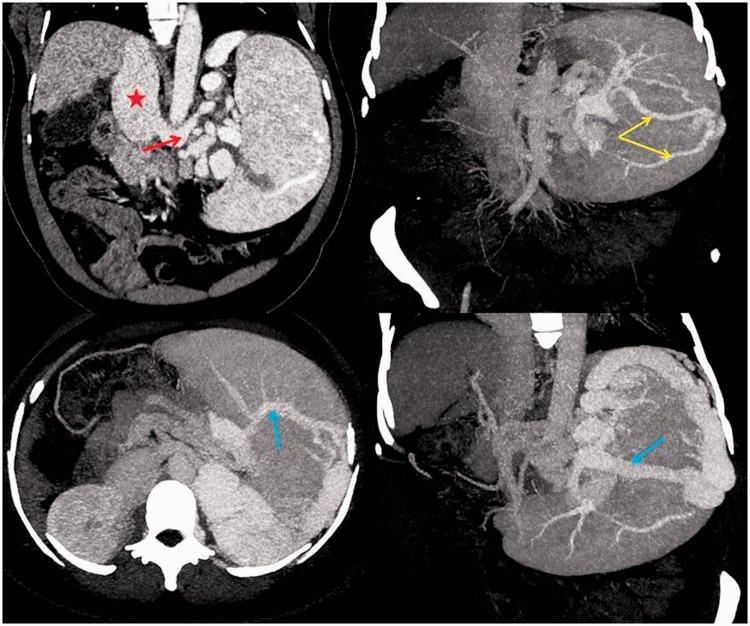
Computed tomography imaging of the abdomen with 3D reconstruction. Clockwise from left: splenorenal shunt from origin of the IVC (red star); left renal vein (red arrow) joining the splenic vein at the hilum; multiple intrasplenic collaterals (yellow arrows); large transplenic shunt (blue arrow); 3D sectional imaging of trans-splenic shunt (blue arrow) with intra-splenic collaterals.
